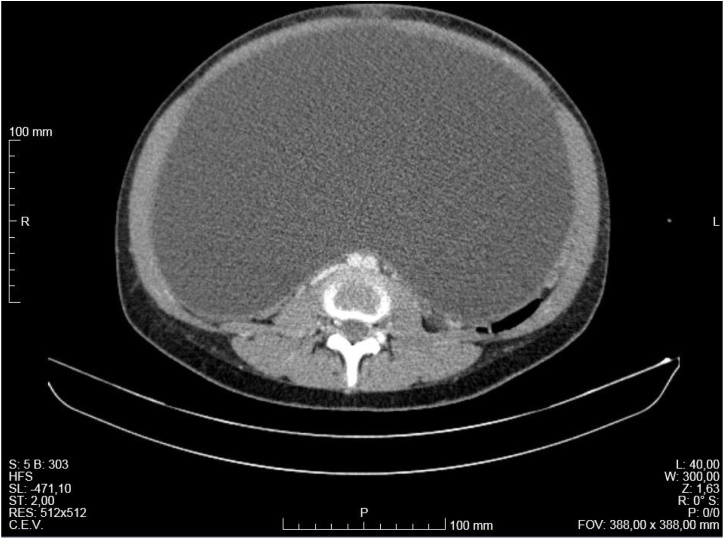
Axial image of abdominal and pelvic CT showing the large size of the cyst with a thin and regular wall, that occupied practically all the abdominal and pelvic cavities, with no contrast-enhanced solid areas, that deviated and collapsed the abdominal and pelvic viscera.
